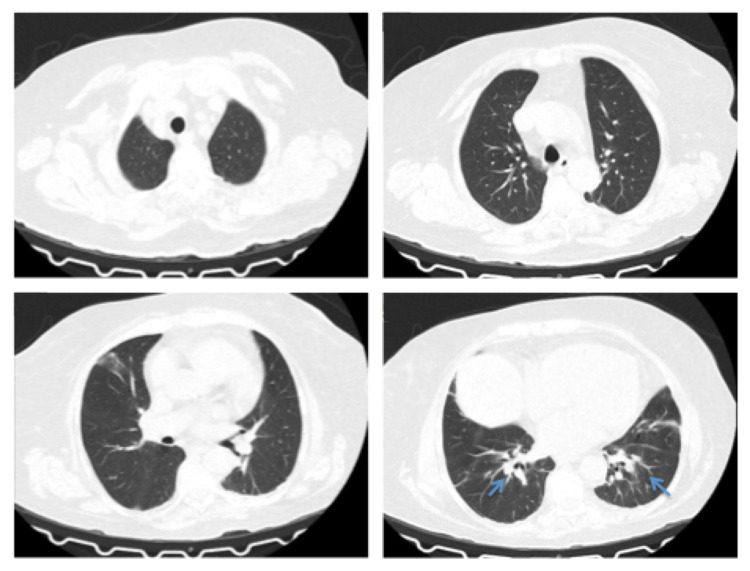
Chest CT at admission. Chest CT scan at admission showed bilateral infiltrates in lower lobes and subpleural in the lingula.
